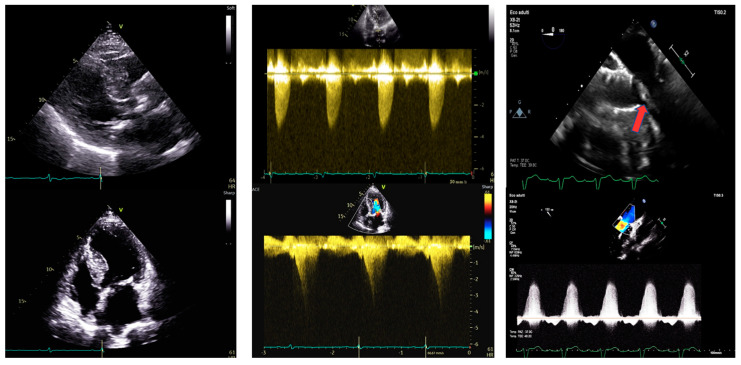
Transthoracic and transesophageal echocardiography in an 87-year-old woman with paradoxical low-flow low-gradient aortic stenosis. Left panel. PLAX view (upper) and 4-chamber view (lower). IVS telediastolic diameter = 20 mm, LVEDVi = 31 mL/m^2^. Central panel. Aortic valve peak velocity of 3.6 m/s (upper) with a mean gradient of 28 mmHg and a dynamic LVOT gradient (lower) of 54 mmHg after the Valsalva maneuver. Right panel after TAVI. SAM of the anterior mitral leaflet (upper, red arrow) by TEE causing severe mitral regurgitation. IVS: interventricular septum. LVEDVi: left ventricular end-diastolic volume indexed. LVOT: left ventricular outflow tract. SAM: systolic anterior motion.
